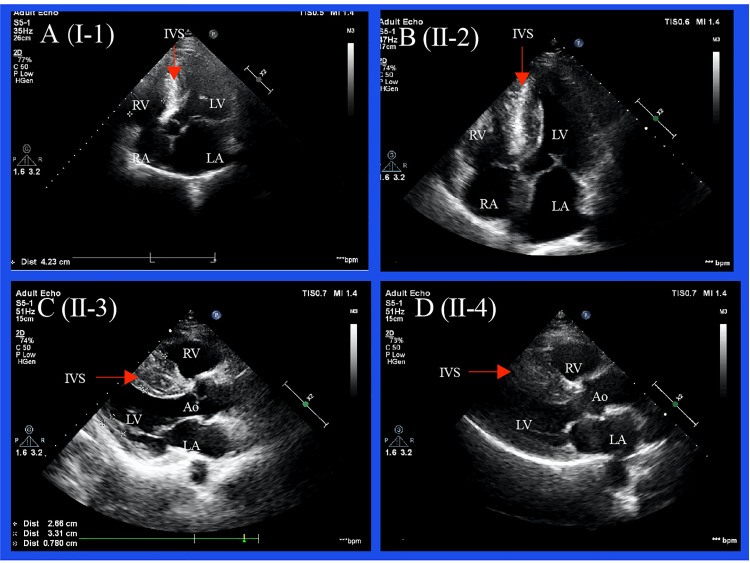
Typical echocardiography of family members. Four chamber views of I-1 and II-2. Parasternal long axis view of II-3 and II-4. LA = left atrium; LV = left ventricle; RA = right atrium; RV = right ventricle; Ao = aorta.
